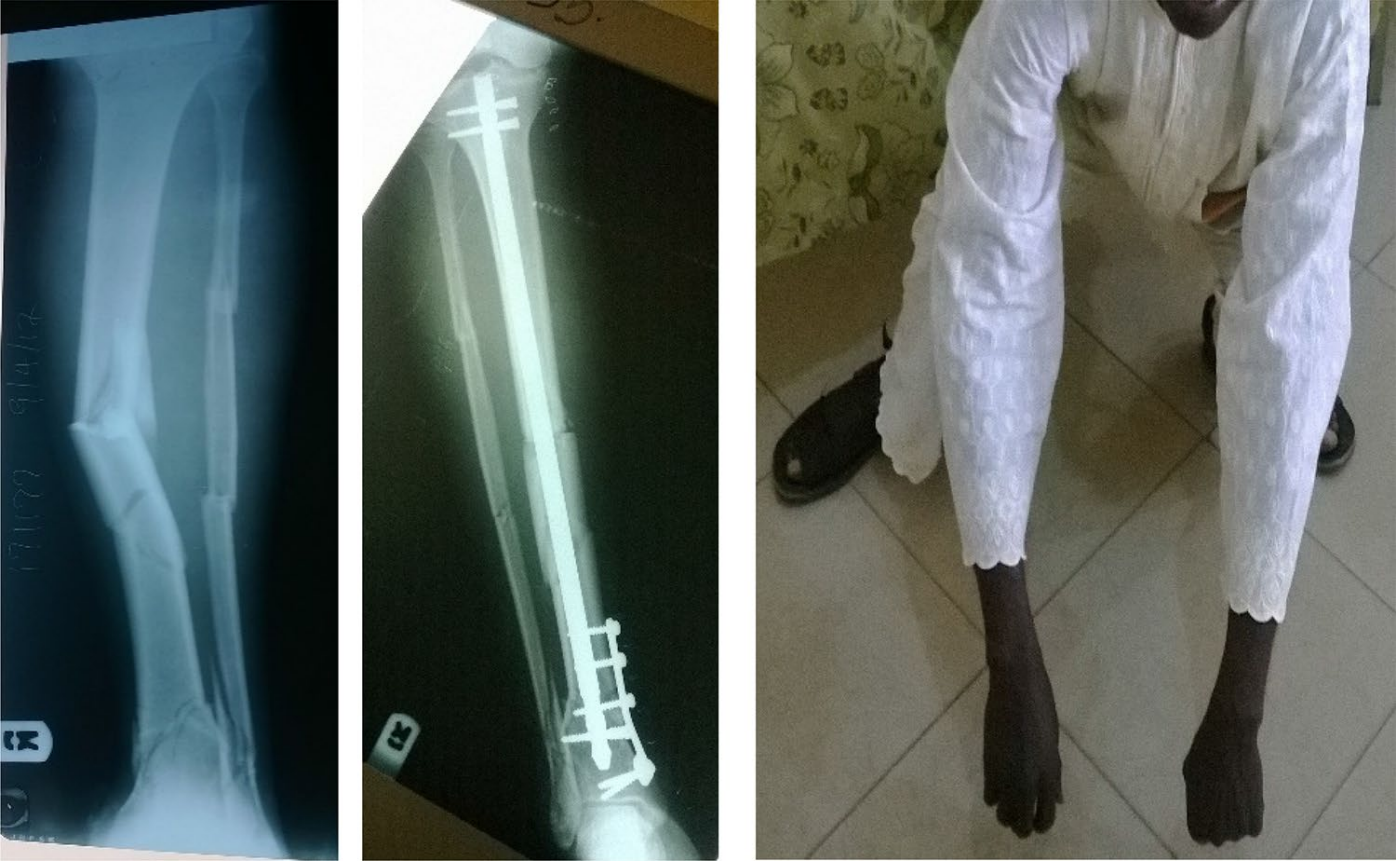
Pre- and post-operative radiographs and “squat and smile” photograph (6-month follow-up) of a 50-year old man who had AO/OTA 32C3 (not shown) and 42C3 fractures. The SIGN nail was combined with a side plate to treat the most distal tibia fracture.
